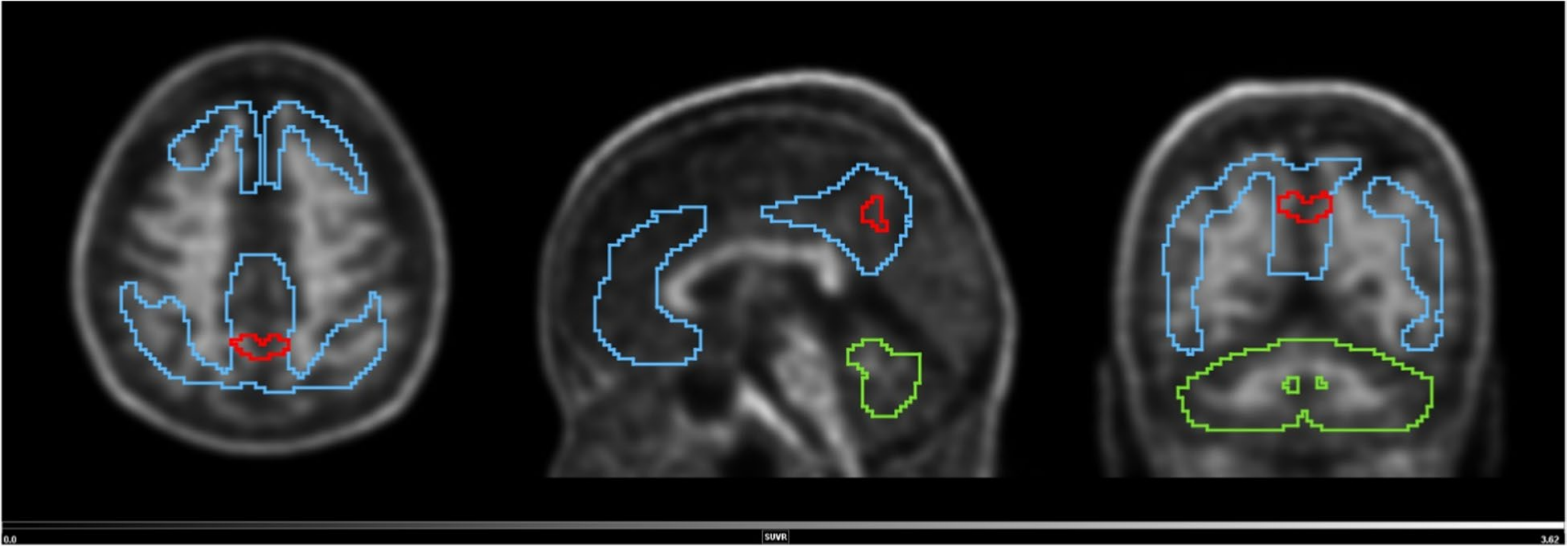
Volume with higher baseline signal for N1 accumulators and N1 non-accumulators of amyloid (red line) overlayed on the scan of an N1 accumulator (MNI space). Grey scale shows SUVR range. Blue line shows the Centiloid target region and the green line shows the reference region (whole cerebellum)
